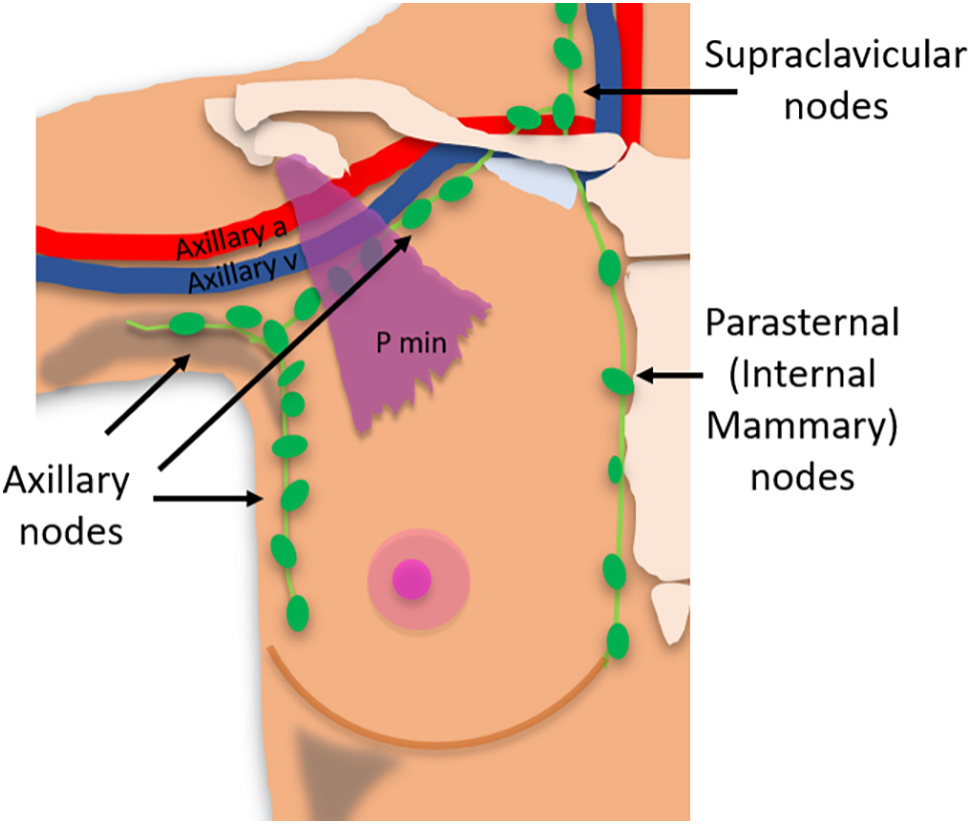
Regional lymph nodes of the breast which include: Intramammary, axillary, parasternal (internal mammary) and supraclavicular lymph nodes.
